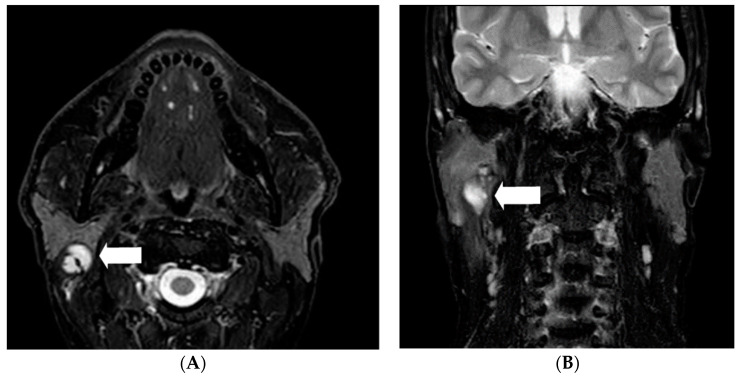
MRI of head and neck, 1.5 tesla magnet. T2 fatsat imaging in axial (A) and coronal (B) orientation. Cystic lobulated tumor in the medial lobe of parotid gland adjacent to the facial nerve (arrow).
